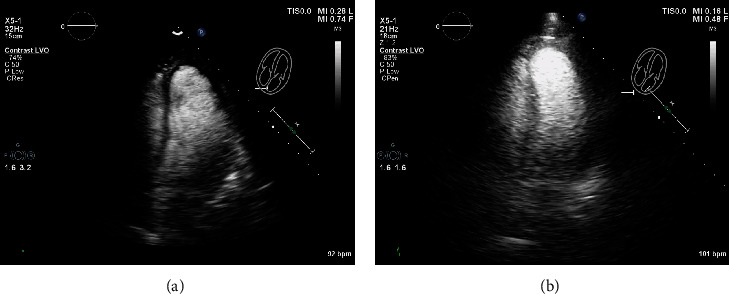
(a–b) Apical 4 chamber view with ultrasound-enhancing agent demonstrating hypokinesis of basal left ventricle and akinesis of all other segments. (b) Apical 4 chamber view with ultrasound-enhancing agent demonstrating recovery.
